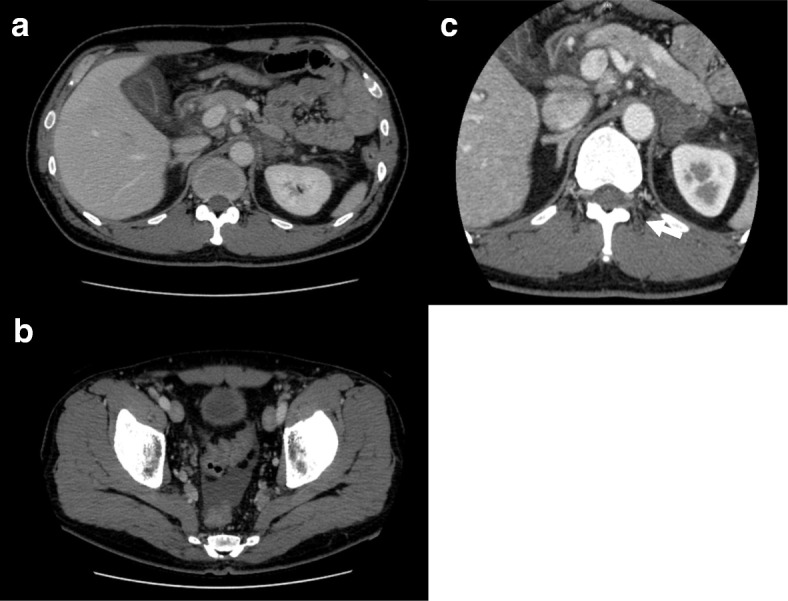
The images of the first contrasted computed tomography scan. a Edema around the gallbladder. b Ascites. c Left adrenal gland without the contrast effect (*arrow*). Organomegaly including hepatosplenomegaly and lymphadenopathy is not seen here
